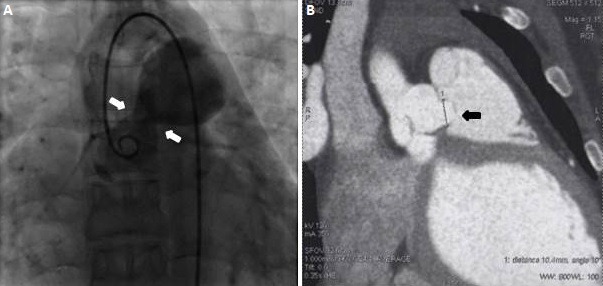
Angiograms (aortography and CT angiography) show ruptured right sinus of Valsalva aneurysm into the right ventricle
